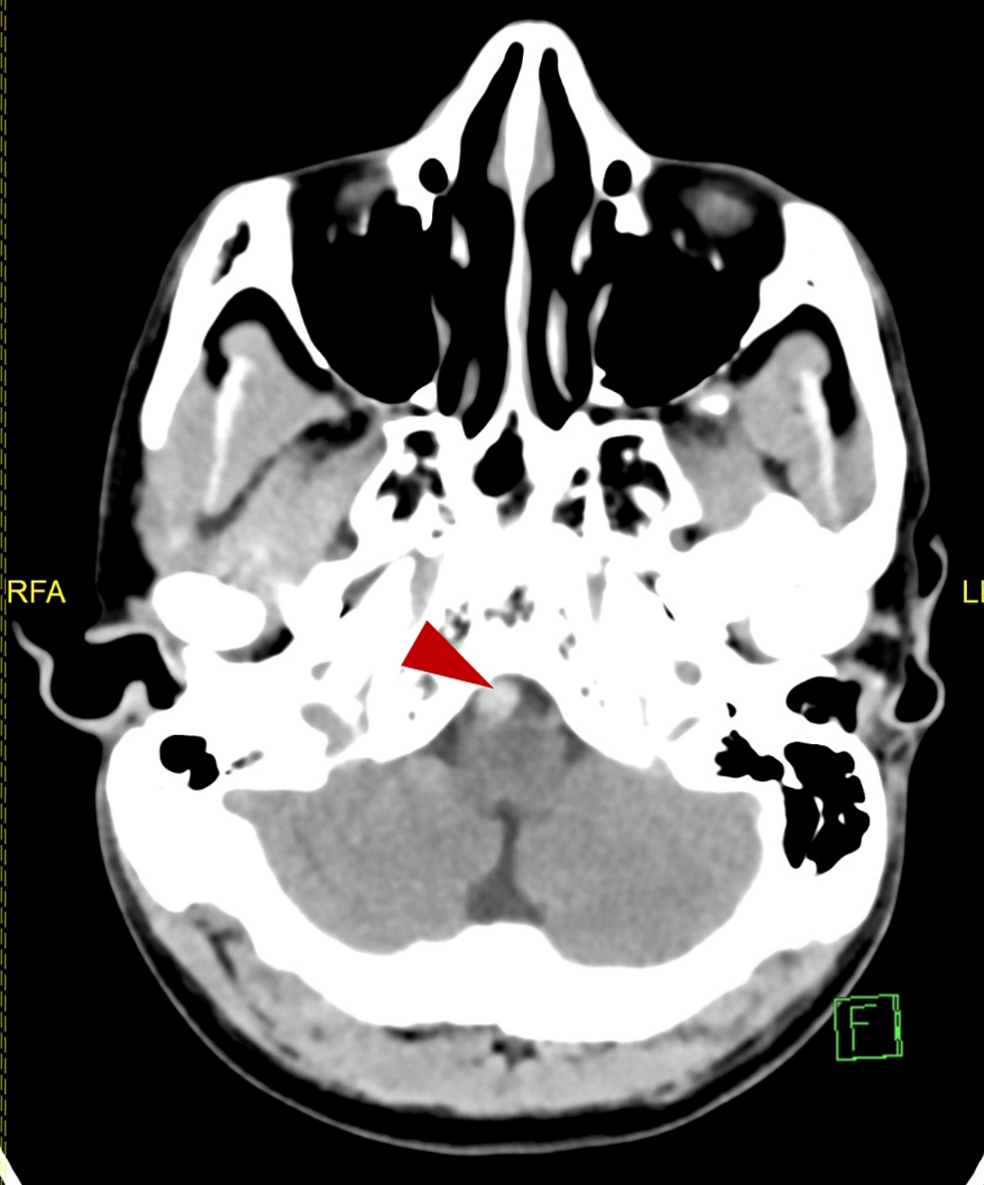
Computed tomography. Arrowhead showing hyperdensity of the right vertebral artery.
